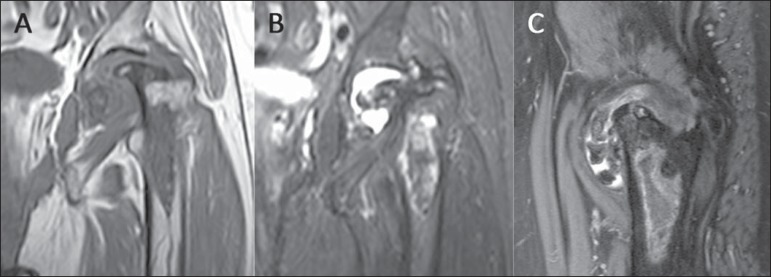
MRI of the same patient on Figure 3. Coronal
